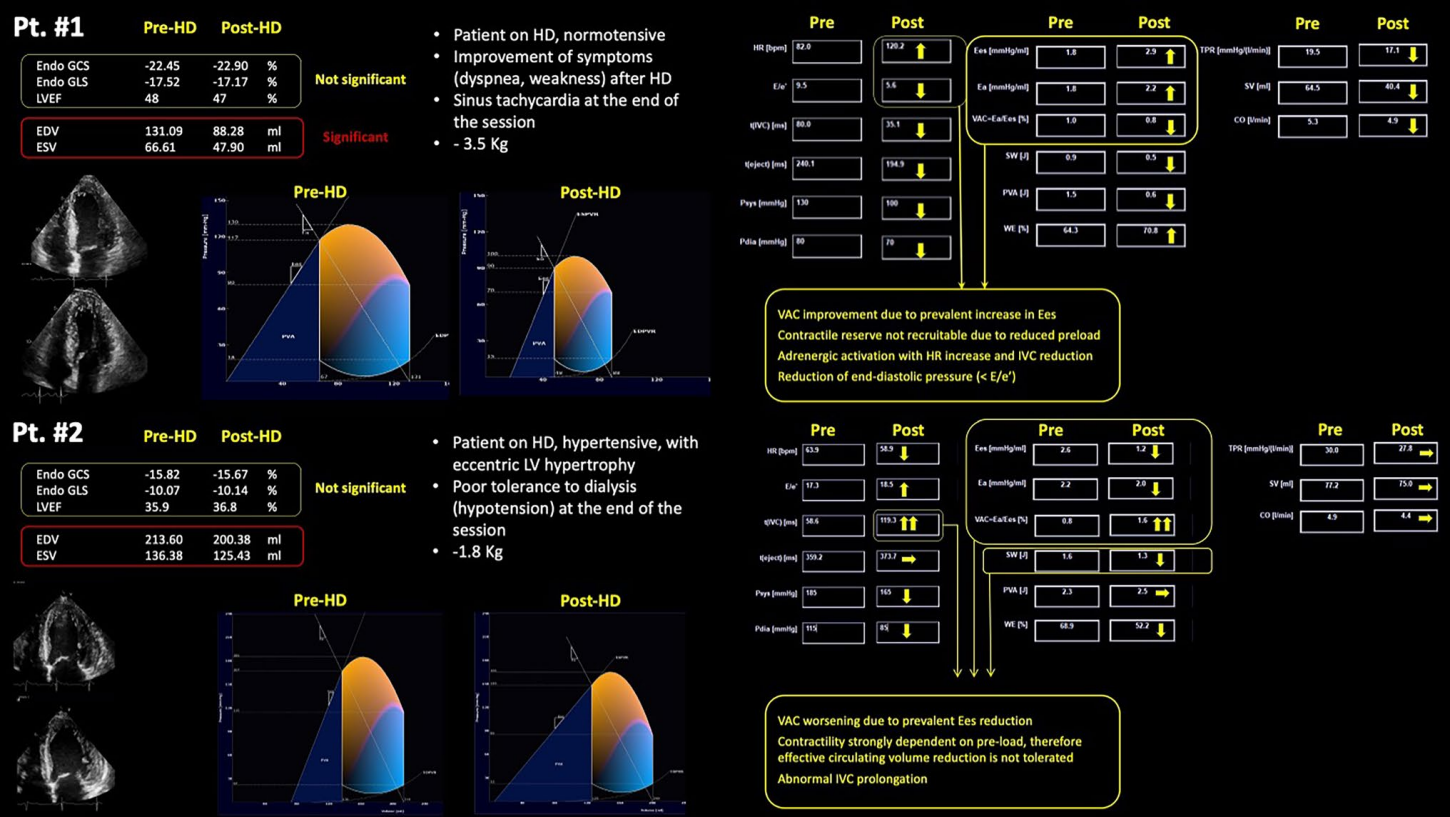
Echocardiographic images of two patient cases with kidney failure on chronic HD (from Salustri et al., [78])
